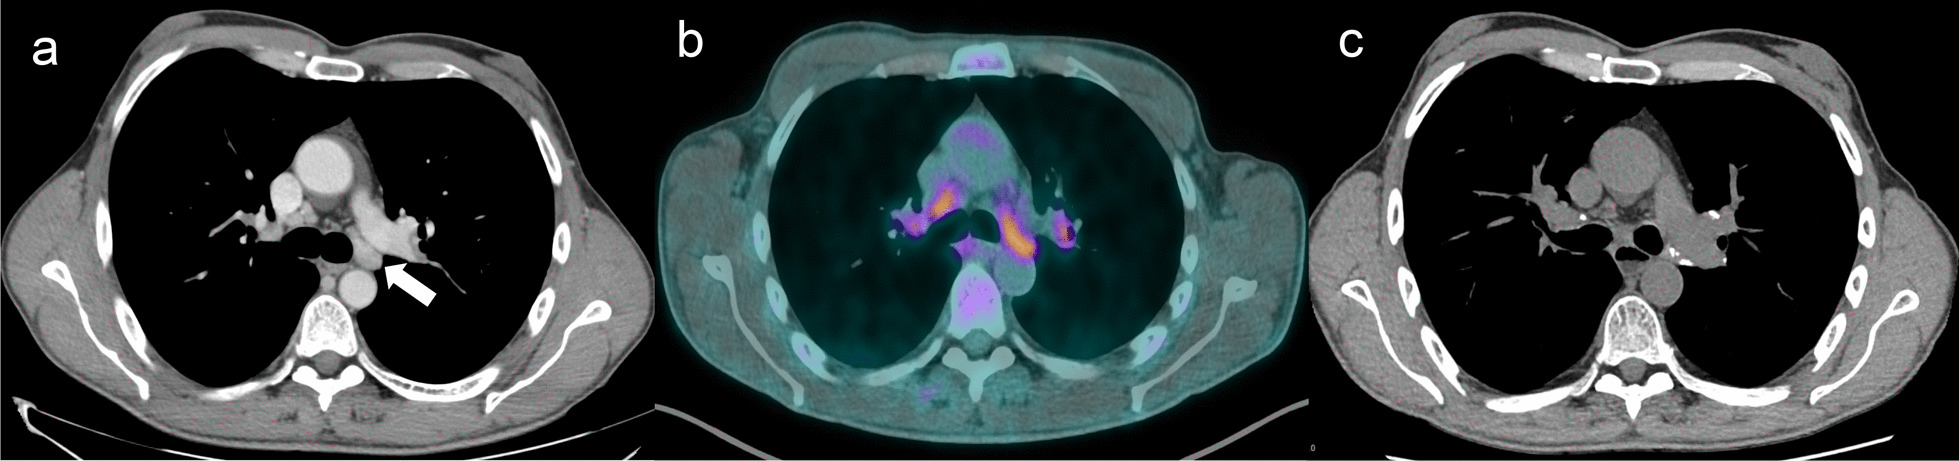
Example of thoracic LAP evolution in one patient exposed to silica. One Thoracic LAP has been revealed in 4L area on first thoracic MDCT assessment (a, white arrow) with an hypermetabolism on PET-CT (b). At 9 months, a spontaneous regression of the thoracic LAP has been highlighted on thoracic MDCT with occurrence of calcifications (c)
